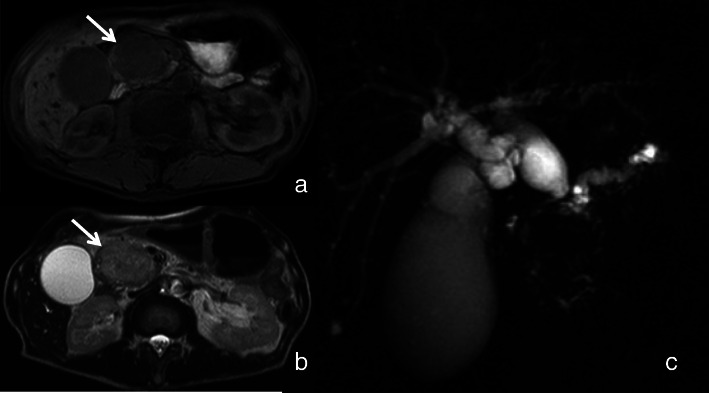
MRI showed a tumor in the pancreatic head showing low intensity on T1-weighted images (a) (arrow) and high-intensity images on T2-weighted images (b) (arrow). MRCP revealed a dilation of the main pancreatic duct from the body to the tail of the pancreas and luminal narrowing of the common bile duct (c)
